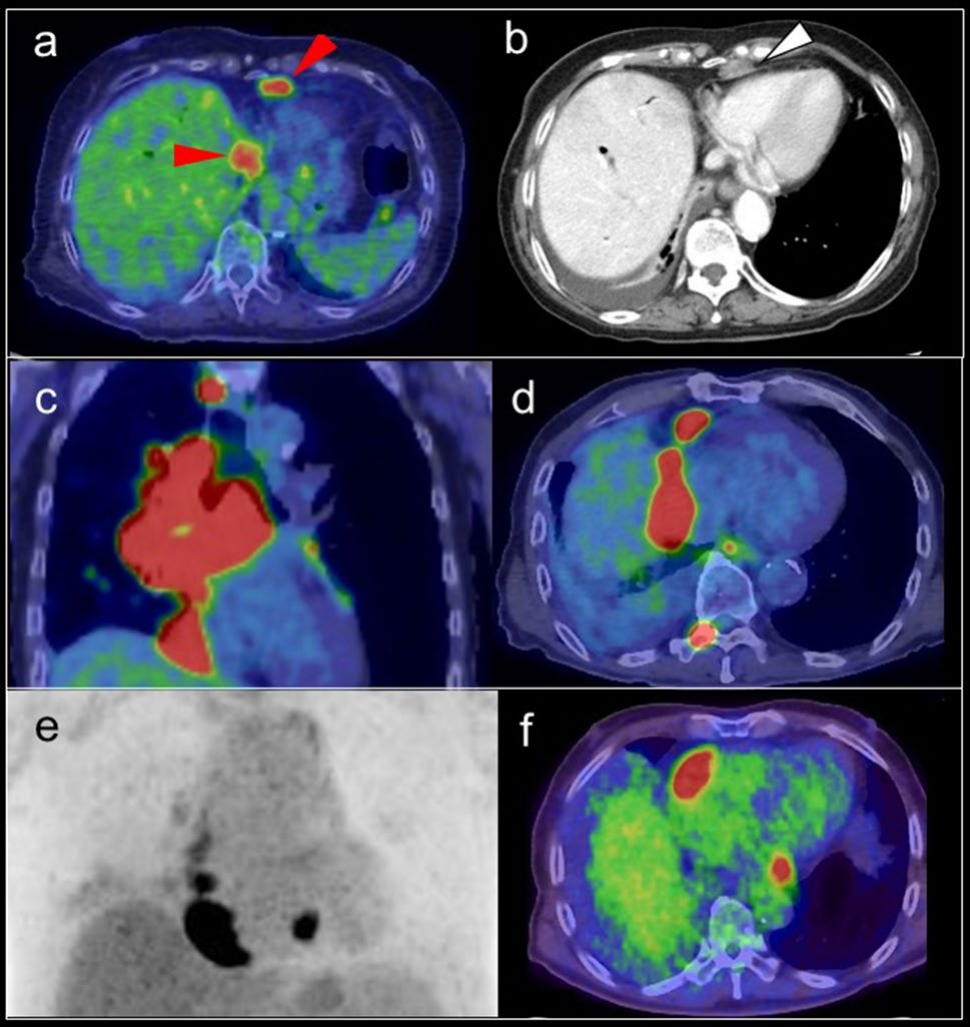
Pericardial lesions. FDG-PET/CT depicted pericardial metastatic lesions (red arrowhead) in patient with gastric cancer (a). Contrast enhanced CT showed just a lesion matched to lesion showing FDG uptake (b). Malignant lymphoma lesion was involved in the pericardium (c, d). Focal FDG uptake in the pericardium was proved pericardial invasion of tuberculosis
